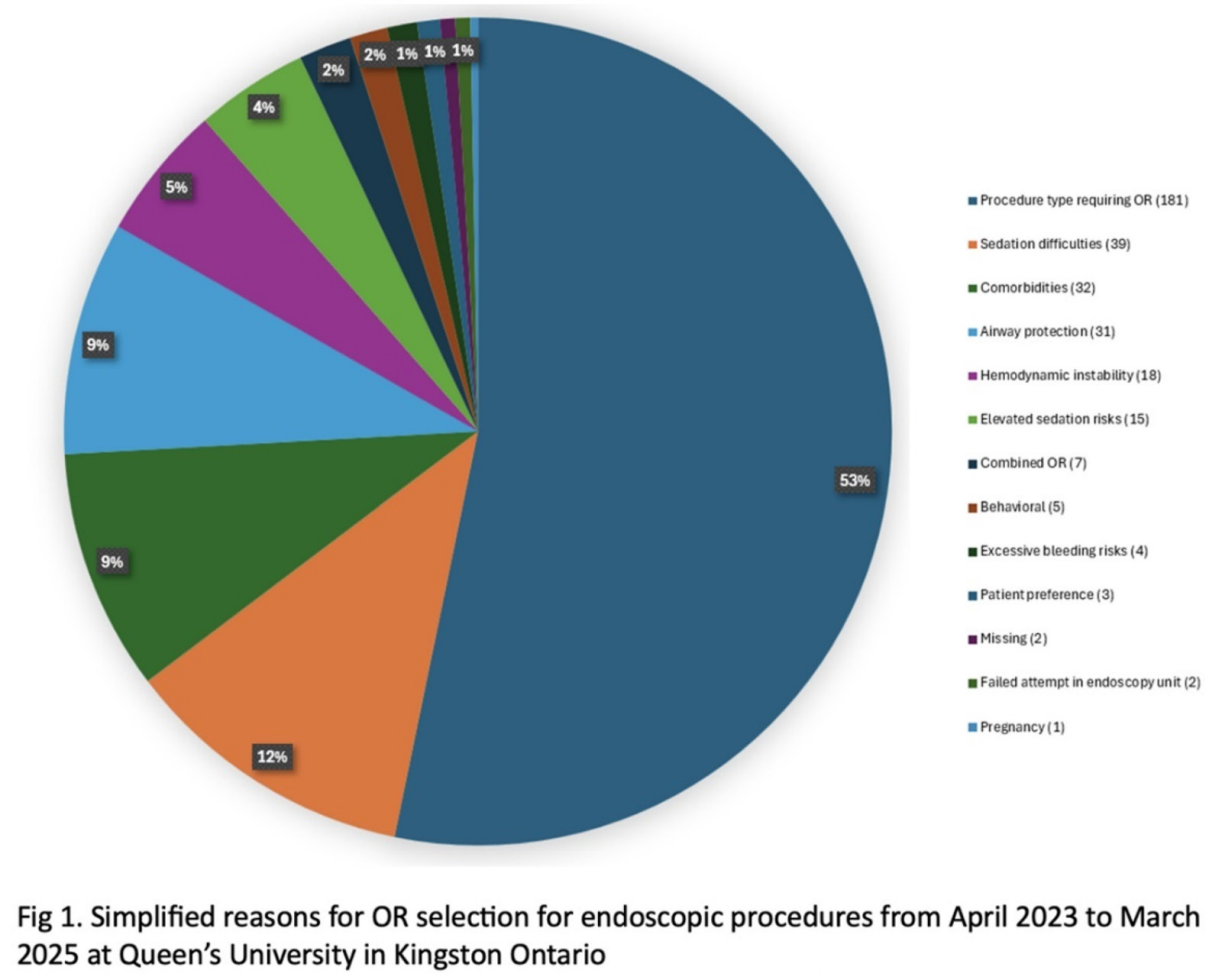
# Poster Session I - A145 RETROSPECTIVE SINGLE CENTER REVIEW OF ENDOSCOPIC PROCEDURES REQUIRING ANESTHEISA ASSISTANCE IN THE OPERATING ROOM
